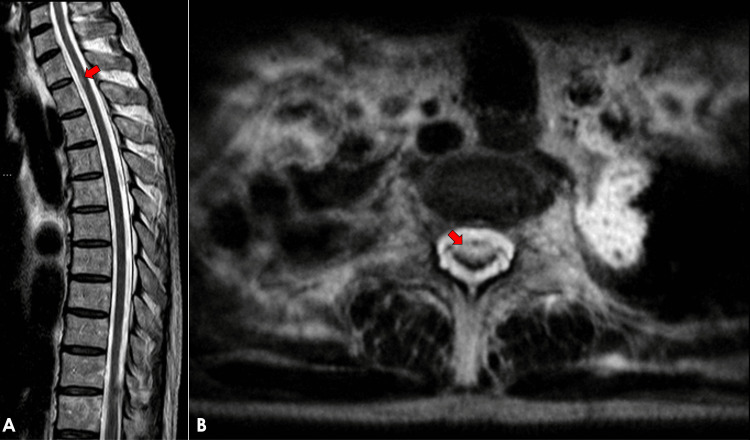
Magnetic resonance imaging of the thoracic spine demonstrating intramedullary spinal cord abnormalities. (A) Sagittal T2-weighted image showing a focal intramedullary hyperintense lesion at the level of the first and second thoracic spinal cord segments (arrow). (B) Axial T2-weighted image at the corresponding level demonstrating the intramedullary hyperintense signal within the spinal cord (arrow).
